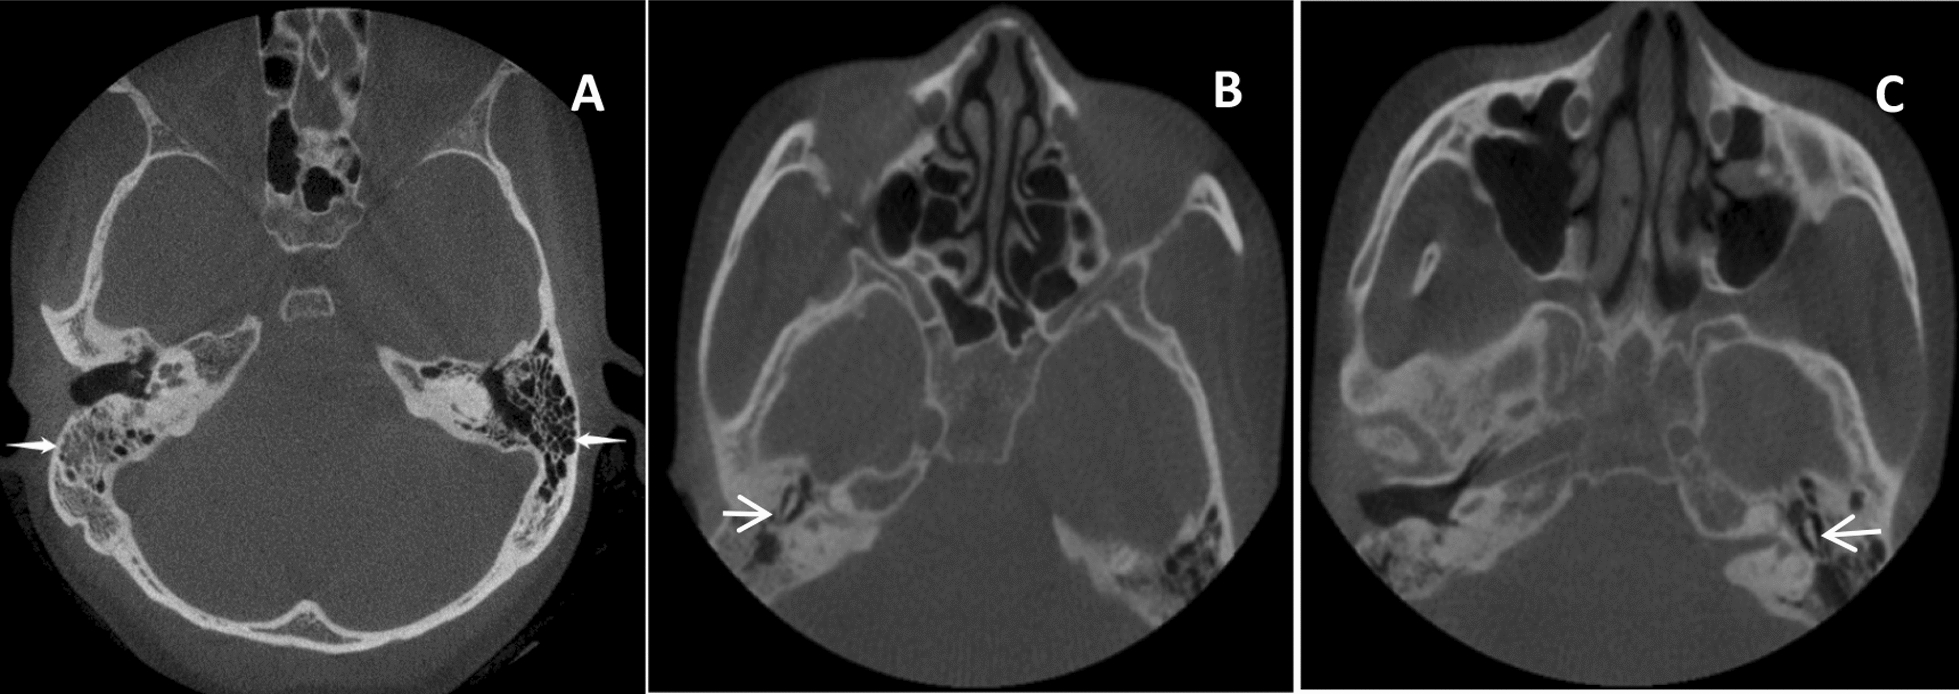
Temporal bone computed tomography findings. A Axial section demonstrating opacification of right mastoid air cells (arrow), with intact contralateral pneumatization. B, C Coronal sections showing unobstructed bilateral mesotympanums (arrows), without evidence of middle ear effusion or ossicular pathologies
